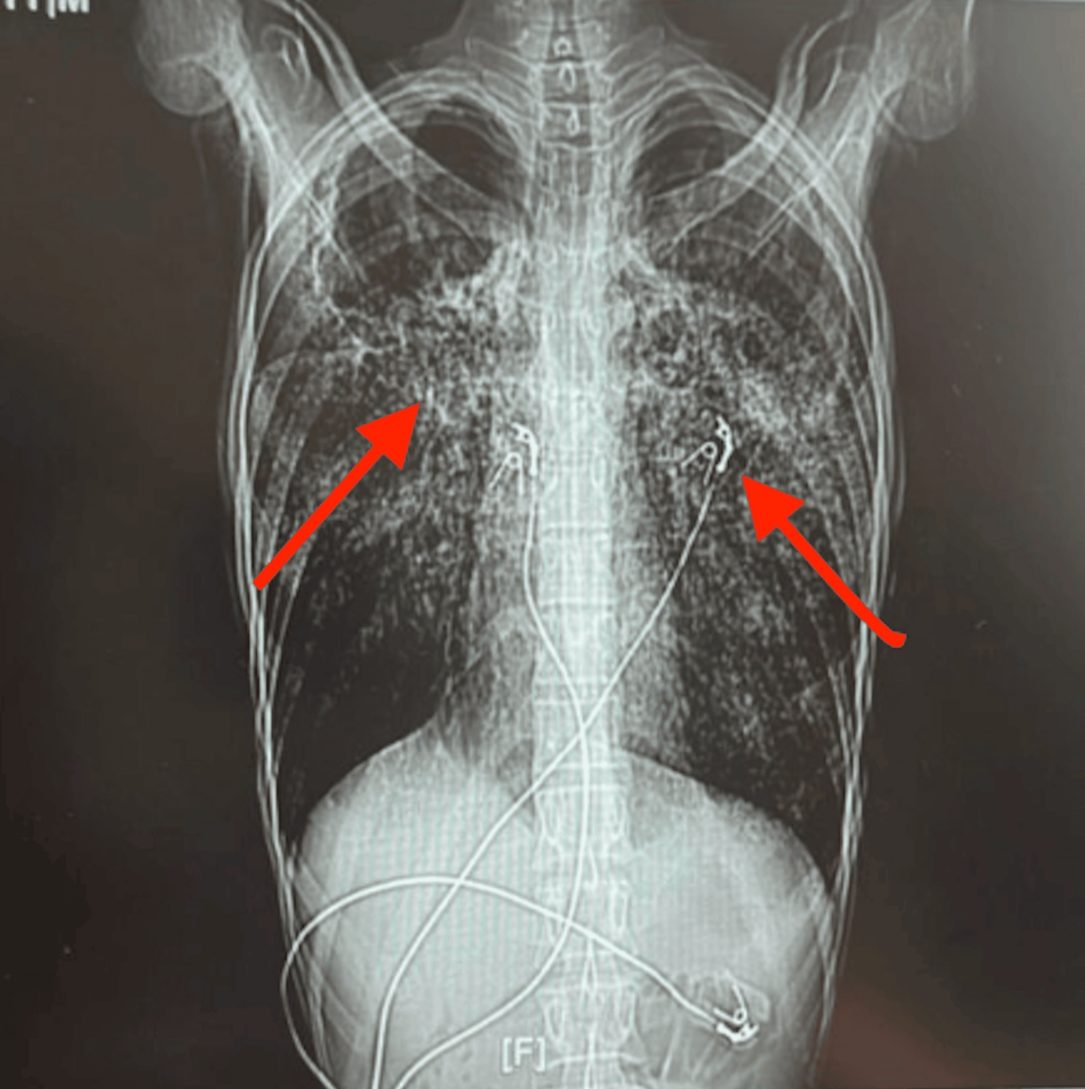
CT scan of the chest and abdomen without contrast. CT scan demonstrating diffuse bilateral micronodular opacities consistent with a miliary pattern of pulmonary tuberculosis (arrows).
